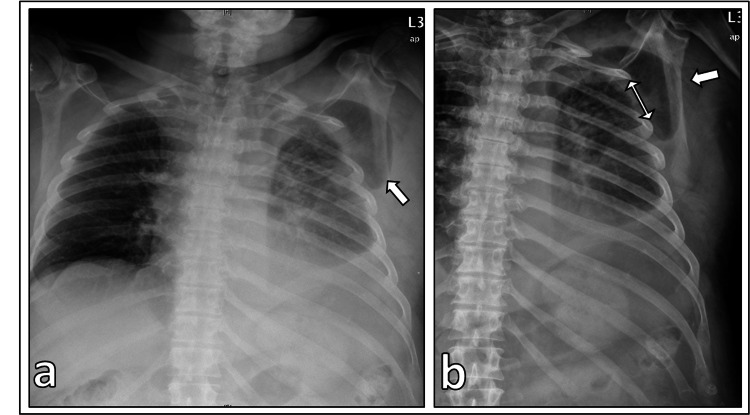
X-ray images X-ray image is showing the extrathoracic lung protrusion (arrow) and the thoracic wall defect between the ribs (double arrow). a. thorax, b. left hemithorax.
